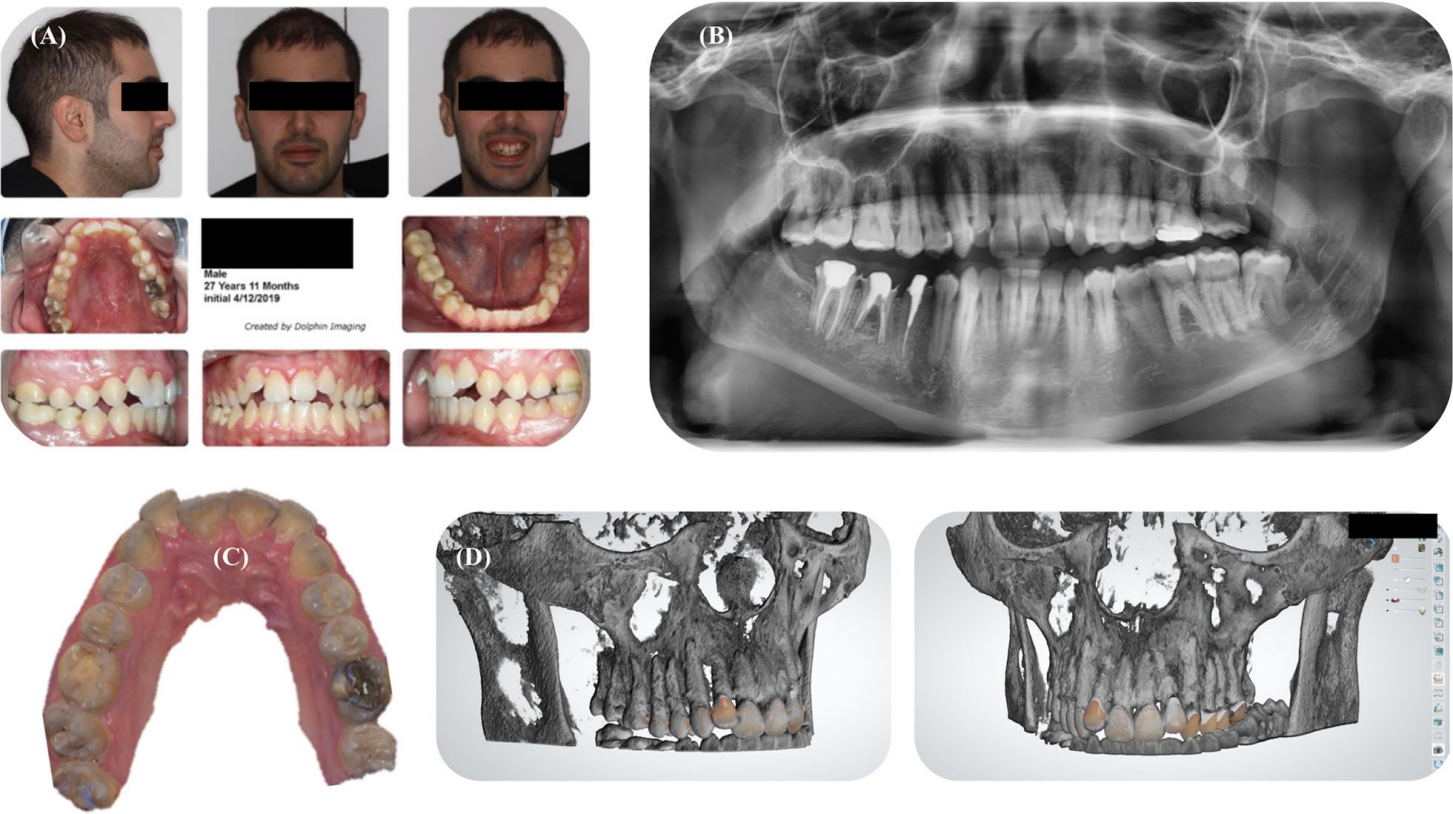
Pretreatment data of the patient. A Intra-oral and extra-oral photographs of the patient. B Panoramic radiograph. C Maxillary intra-oral scan. D Superimposition of the CBCT and the impression using OrthoAnalyzer ™ software
